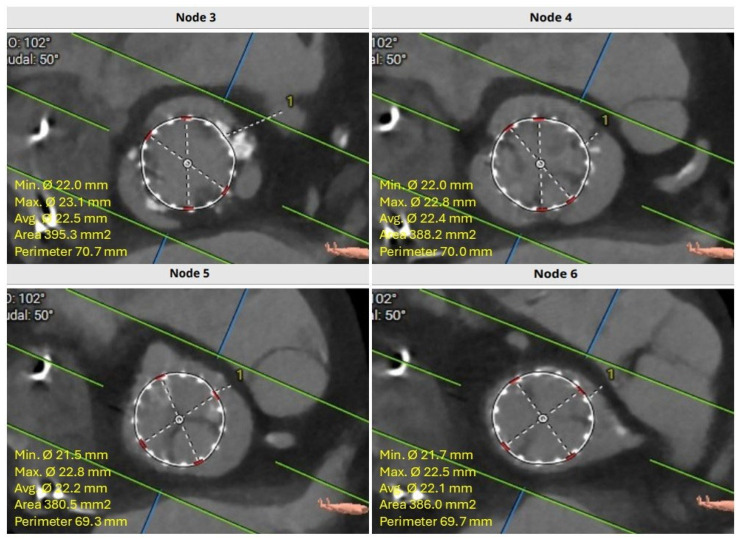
Cardiac CTA demonstrating index THV stent frame dimensions at multiple levels. CTA, computed tomography angiography; THV, transcatheter heart valve.
